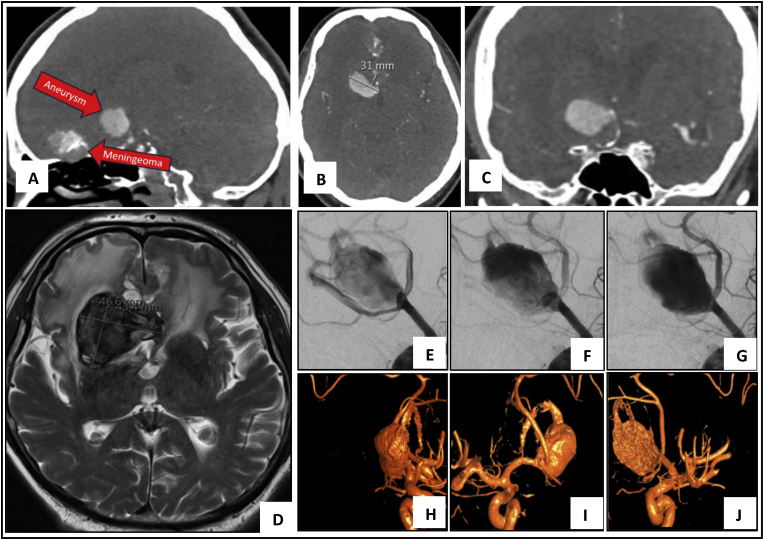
Case two.* Preoperative Imaging – CT Angiography (A) Sagittal cut demonstrating both the aneurysm and meningioma. (B) Axial cut (C) Coronal cut. MR Scan revealing a supergiant intracranial aneurysm (50 mm) with large perifocal edema (D). Digital Subtraction Angiography (DSA) images along with 3D-DSA Reconstruction Images (E–J).
